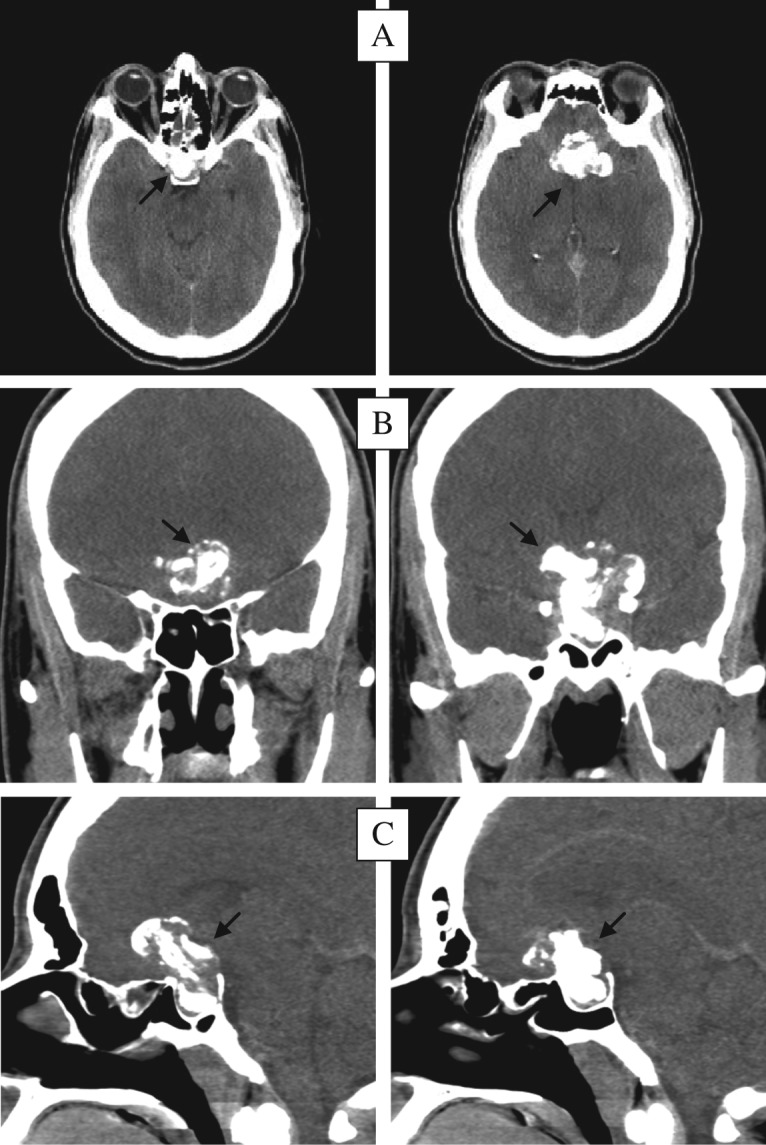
Axial (A), coronal (B) and sagittal (C) CT head images showing the pituitary adenoma with suprasellar and parasellar extension and extensive calcification (black arrows).
